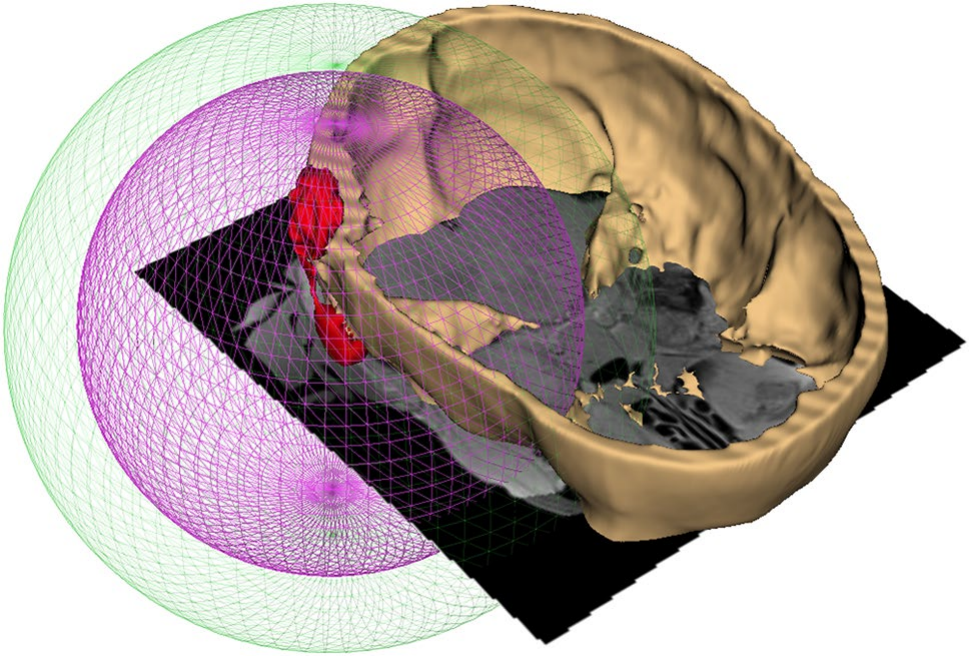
Three-dimensional visualization of simplified artifact dimensions on the registered MRI-CT images. The green sphere represents the artifact extent without the artifact reduction sequence (radius = 9 cm). The purple sphere shows the artifact extent when the SEMAC-VAT WARP sequence is applied (radius = 7 cm). The spheres are centered around the implant (shown in red) placed in the mastoid position
